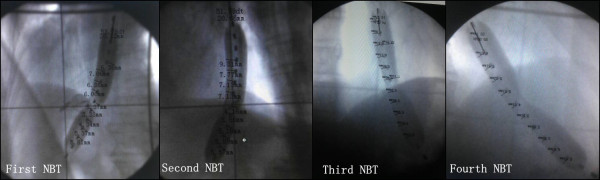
An X-ray image was taken while the applicator and the source were both inserted into the esophagus of a patient. The water balloon can clearly be seen as it is filled with an X-ray contrast agent. The dose was prescribed to the reference point, which was located 10 mm from the center point of the source capsule in the transverse direction. From the first to fourth NBT, the shape of the tumor can be clearly identified.
